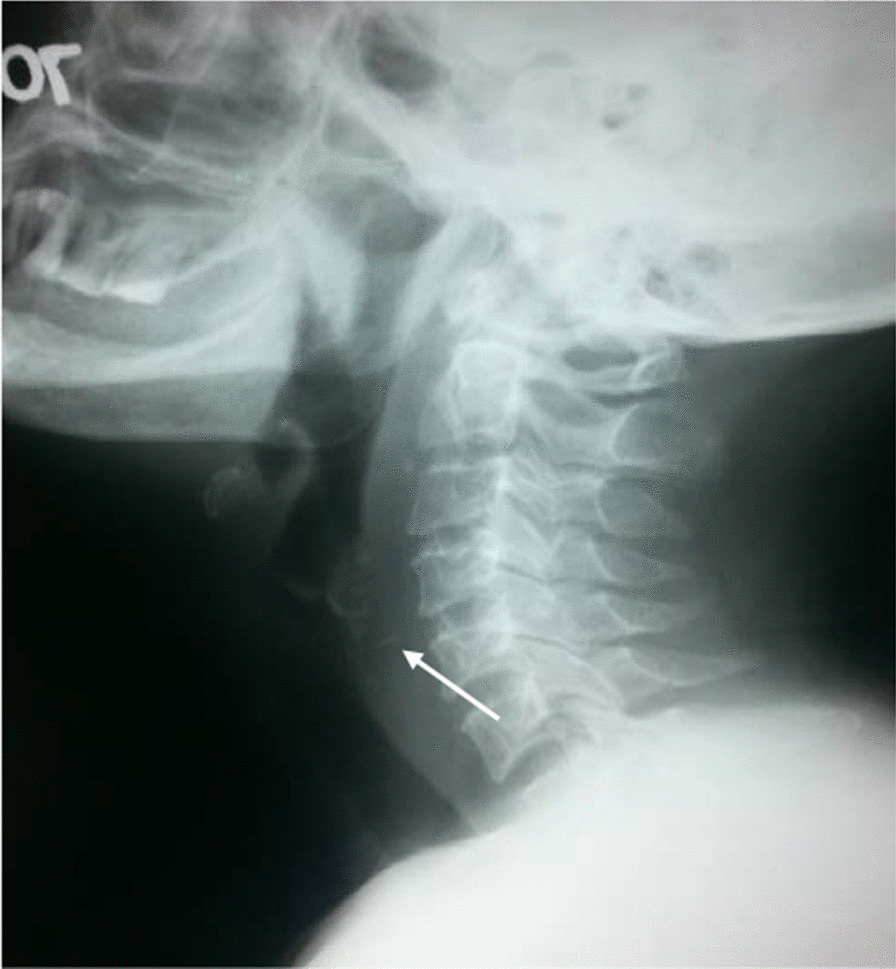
X-ray of the neck. Arrow pointing the fish bone in the X-Ray
